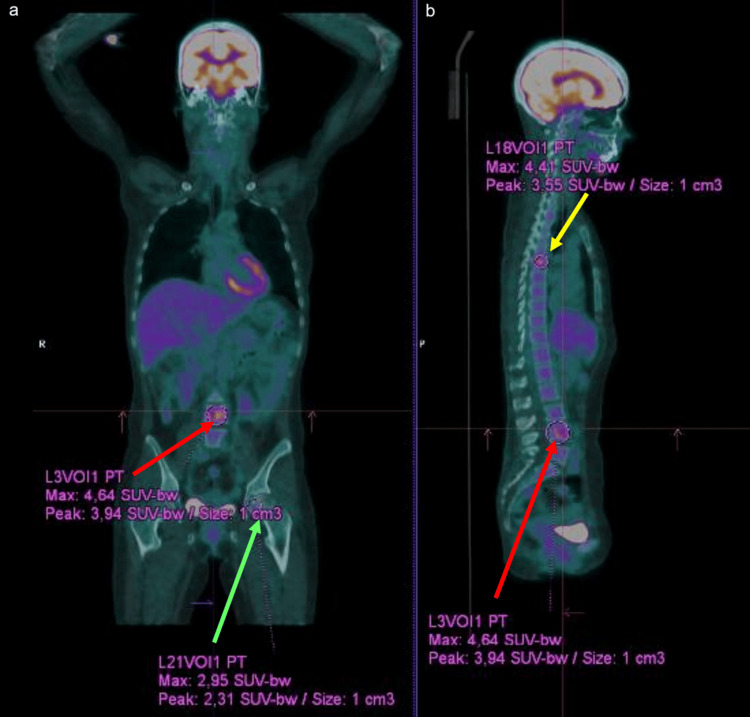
18F-FDG PET/CT with coronal (a) and sagittal (b) views demonstrating hypermetabolic osteolytic lesions involving the T6 (yellow arrow) and L4 vertebral bodies (red arrows), as well as in the left femoral head (green arrow), with SUVmax values ranging from 2.95 to 4.64. fluorodeoxyglucose, PET/CT: positron emission tomography/computed tomography, SUVmax: maximum standard uptake value.
